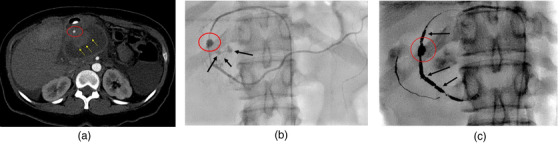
Computed tomography (CT) angiogram with angioembolization of pseudoaneurysm involving gastroduodenal artery. (a) CT angiogram showing gastroduodenal artery pseudoaneurysm (red circle) with surrounding hematoma (yellow arrows). (b) Angiogram showing pseudoaneurysm (red circle) with active extravasation into the pseudocyst (black arrows). (c) Coils packing the pseudoaneurysm (red circle) and gastroduodenal artery (black arrows)
